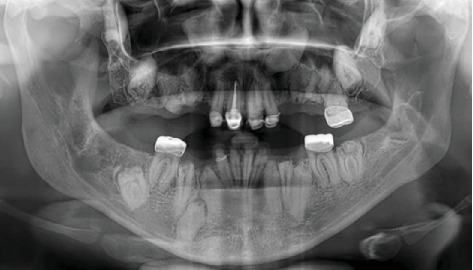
Panoramic radiography. Multiple impacted teeth and “ghost teeth” present.
